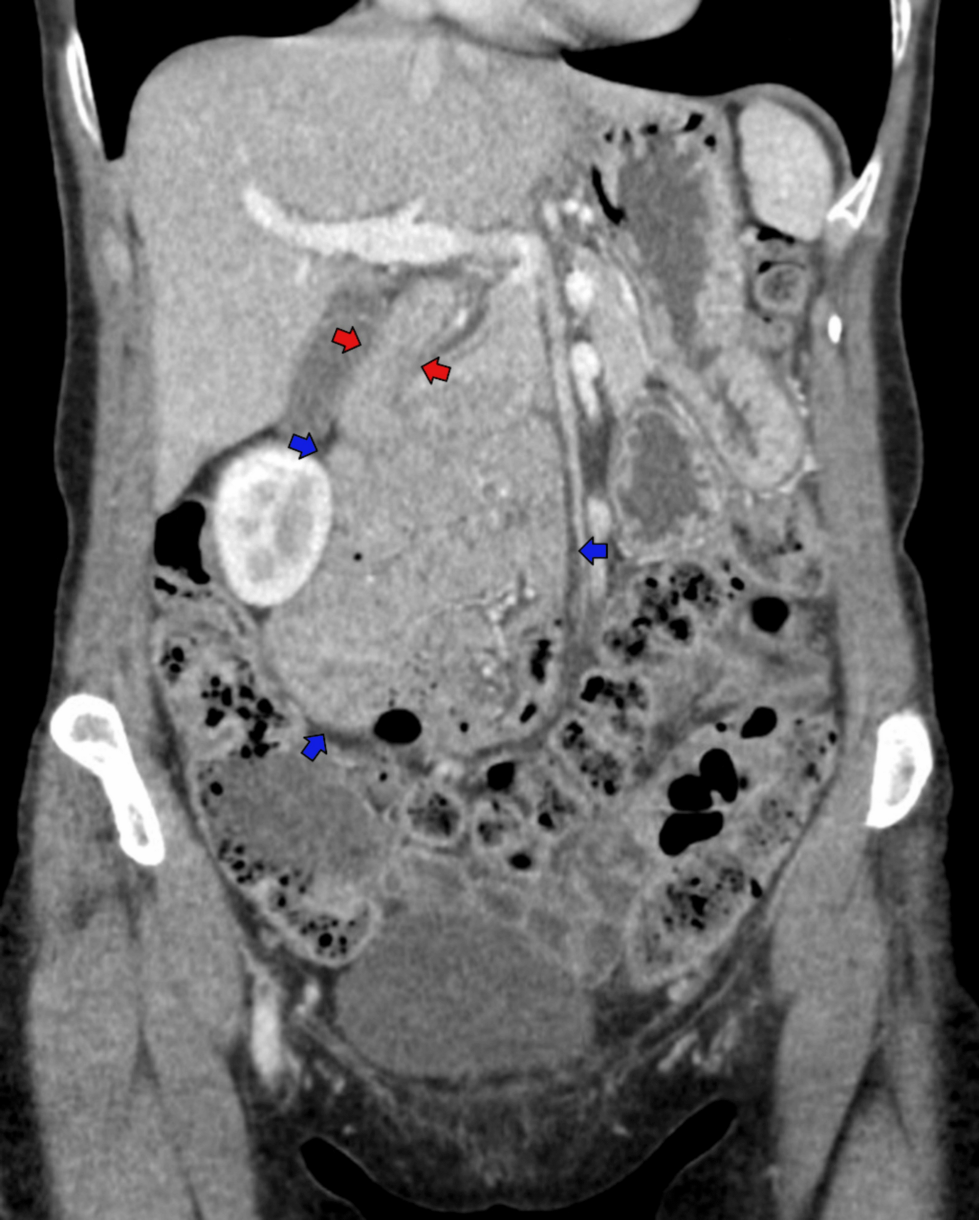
Coronal CT of the abdomen and pelvis demonstrating inferior trajectory of the duodenum and a lack of duodenal sweep (red arrows) and herniation of the majority of the small bowel into right upper quadrant (blue arrows)
